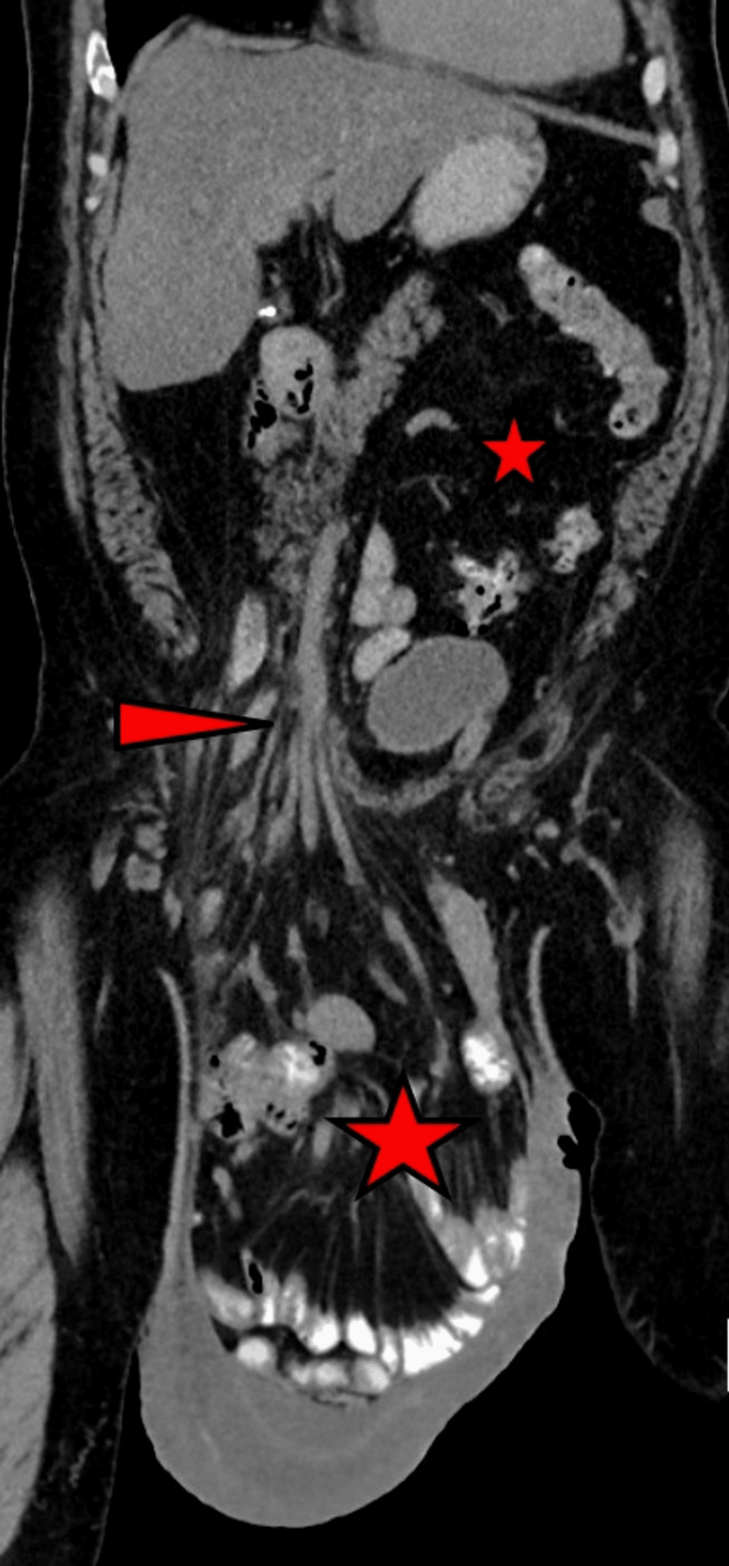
Preoperative computed tomography. Arrowhead shows inguinal canal with herniated intestine and mesenteric root; small star shows abdominal cavity, with almost no small intestine left; and large star shows small intestine in the giant hernia sac.
